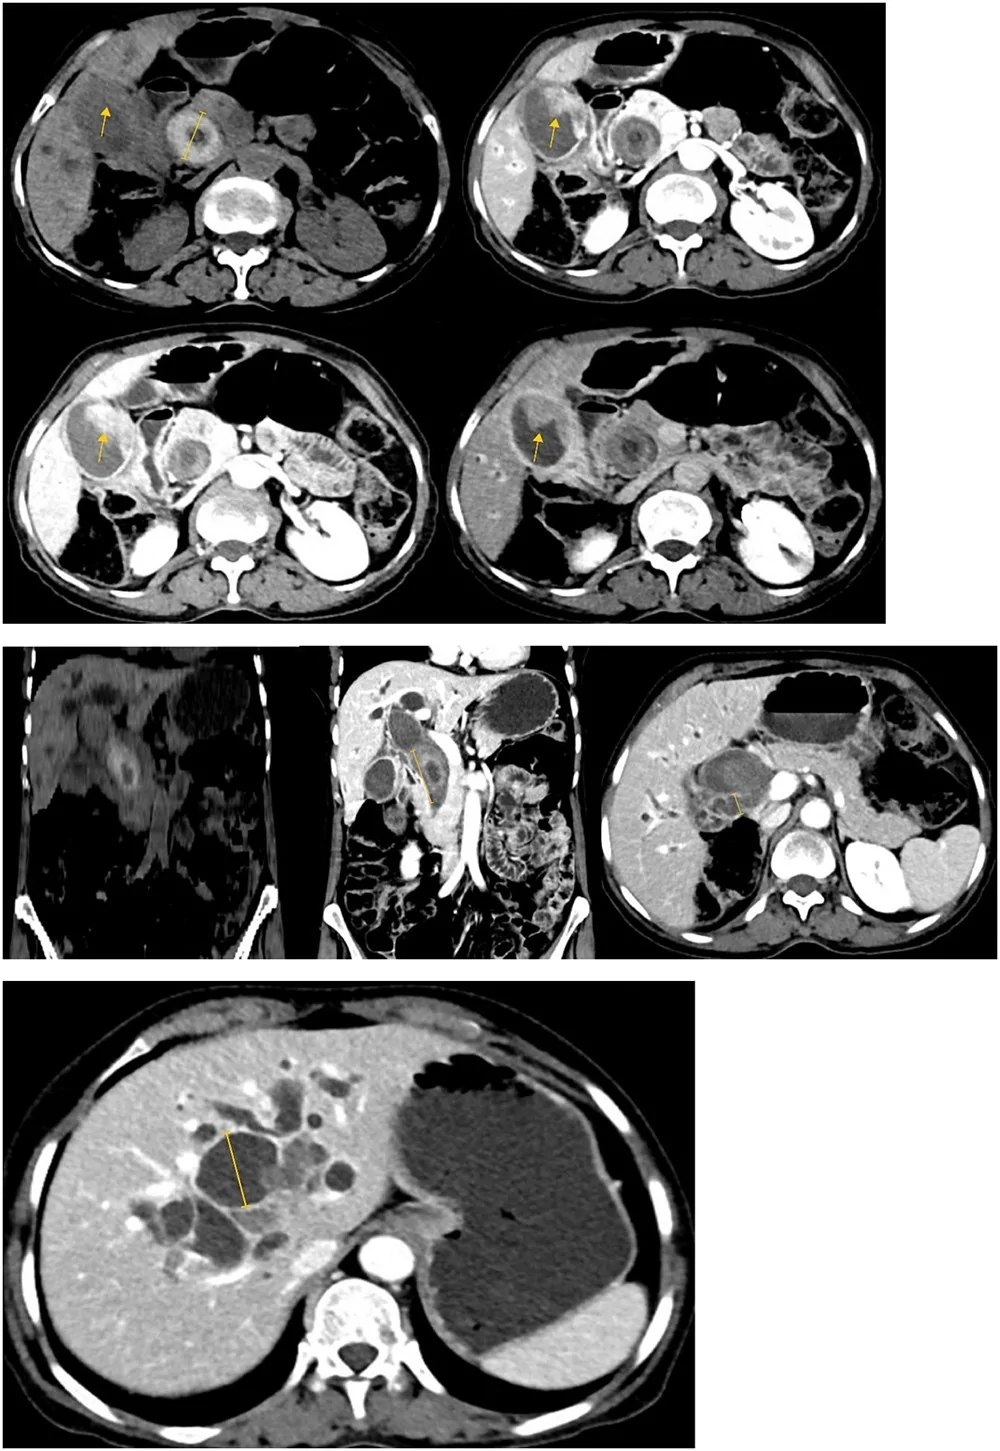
Contrast enhanced CT scan showing gall bladder wall thickening (short yellow arrow) and CBD stone (yellow caliper in the axial and coronal section).
